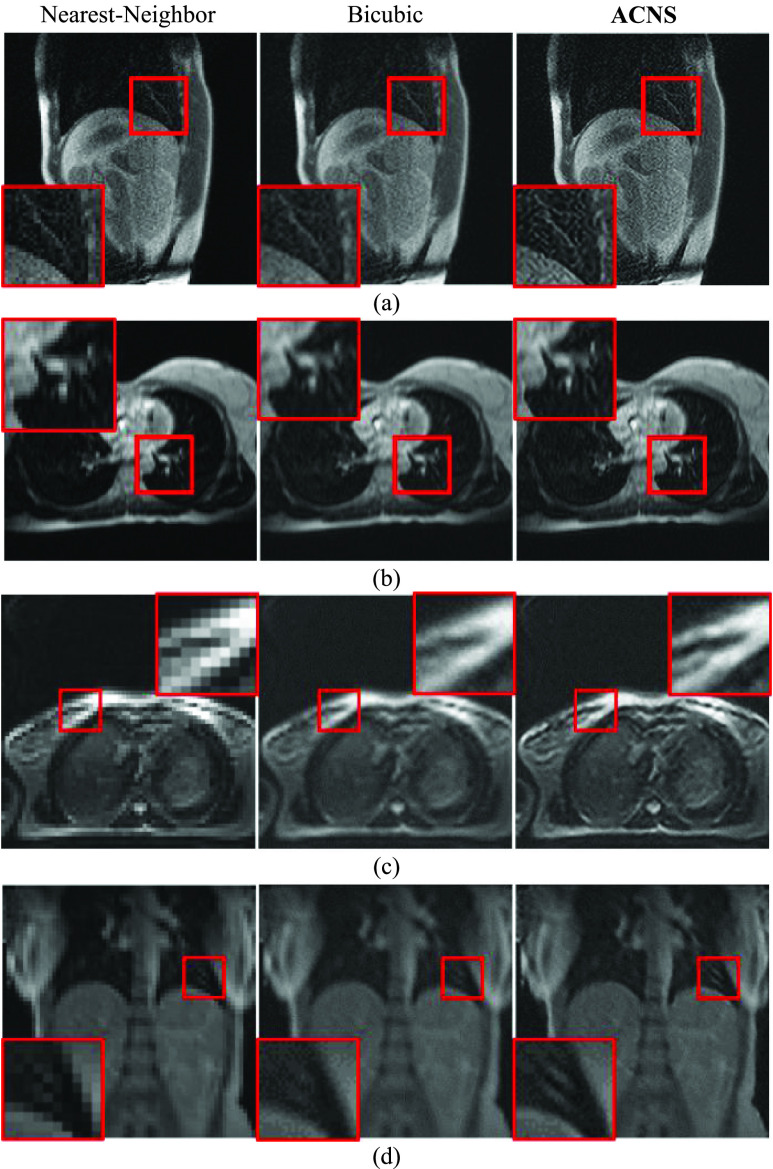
MRIs enlarged by ACNS: (a) Volunteer1, (b) Volunteer2, (c) Volunteer3, and (d) Volunteer4 datasets. Each MRI was randomly selected. The first and second columns are the original MRIs magnified by nearest-neighbor interpolation and bicubic upsampling, and the third column is the resulting MRIs from ACNS, at a }{}$f$ of 3. We magnified the area in the red square to show more details.
